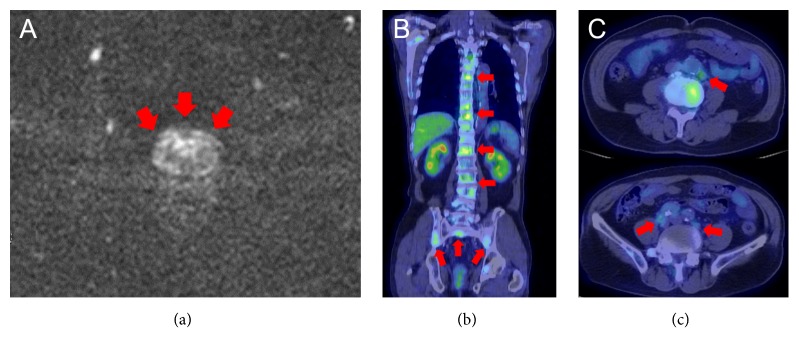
Image findings at initial diagnosis. Pelvic MRI (DWI) (a) showed extensive high signal of the prostate. FDG-PET/CT revealed multiple spine and pelvic bone (b) and para-aortic and pelvic lymph node metastases (c). MRI, magnetic resonance imaging; DWI, diffusion-weighted imaging; FDG-PET/CT, fluorodeoxyglucose-positron emission tomography/computed tomography.
